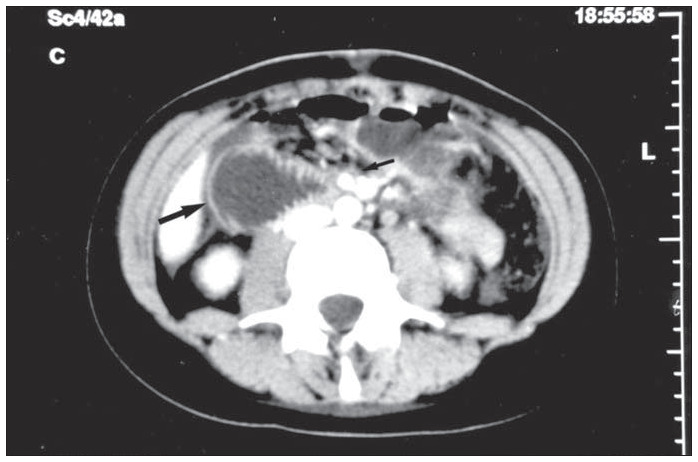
Abdominal computed tomography in the early postoperative period showing duodenal distension with signs of forced contraction (large arrow) and abrupt obstruction at the level of the aortomesenteric constriction (small arrow).
